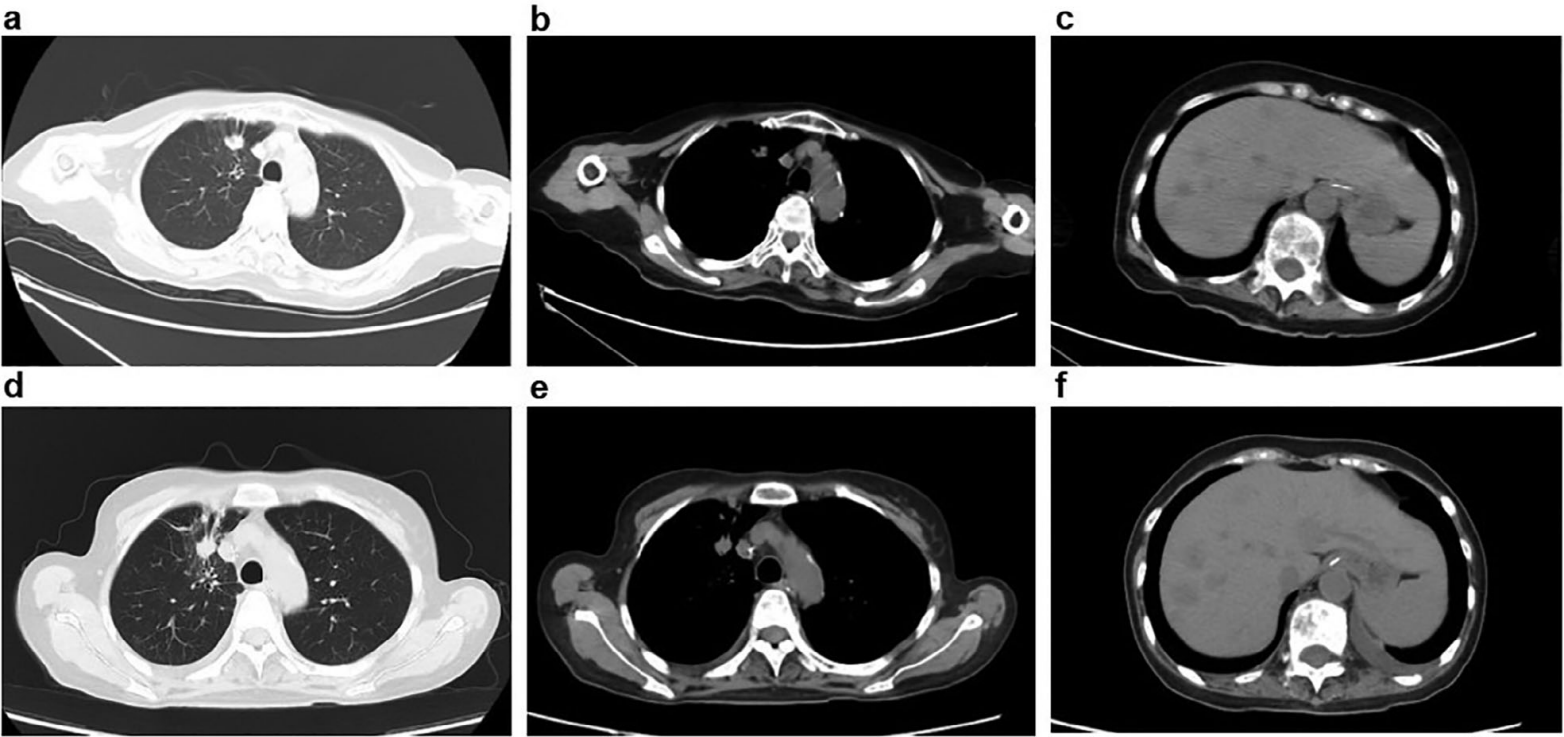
Chest and abdominal radiograph of the patient. (a, b, c) Chest abdominal computed tomography (CT) scan on 6 August 2019, the size of the target lesion 1.2 cm × 1.0 cm, liver metastasis, after one cycle of anlotinib; (d, e, f) chest and abdominal CT scan on 16 October 2019, the size of the target lesion 1.3 cm × 1.1 cm, liver metastasis, after two months of treatment of pyrotinib.
